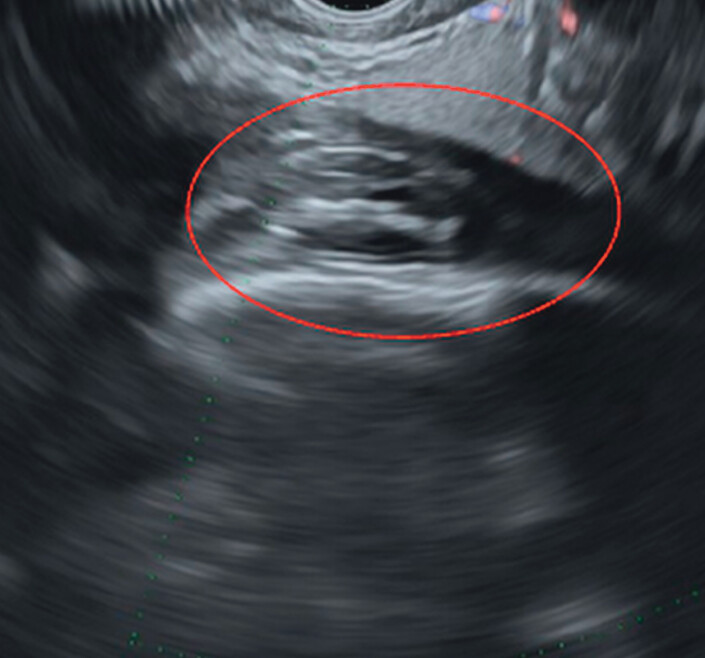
An endoscopic ultrasound view of the gastric conduit lumen (red
